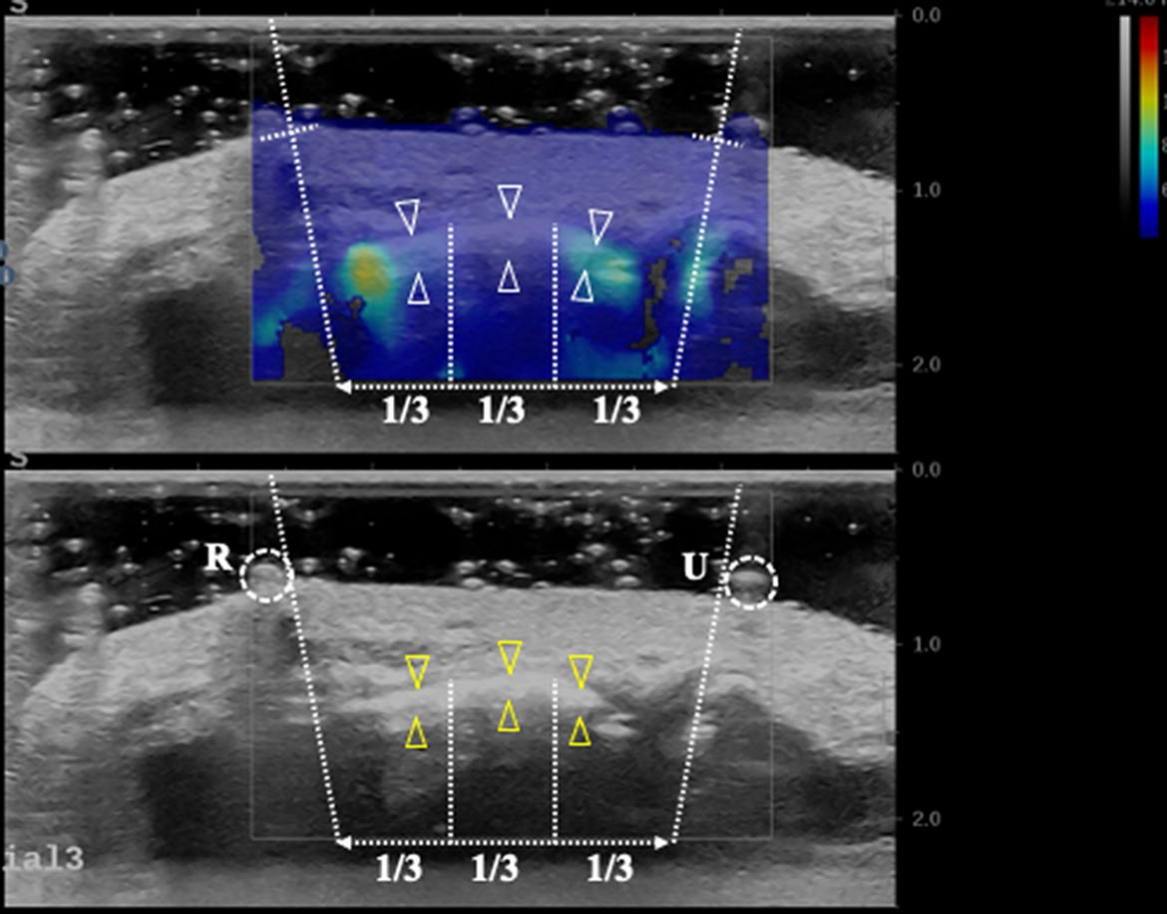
Representative ultrasound images with the outlined middle 1/3 of the transverse carpal ligament. The TCL was labeled by the triangles indicating its volar and dorsal boundaries. The circles indicated as R and U are the two opaque skin markers at radial and ulnar used to approximately defined endpoints of the TCL
